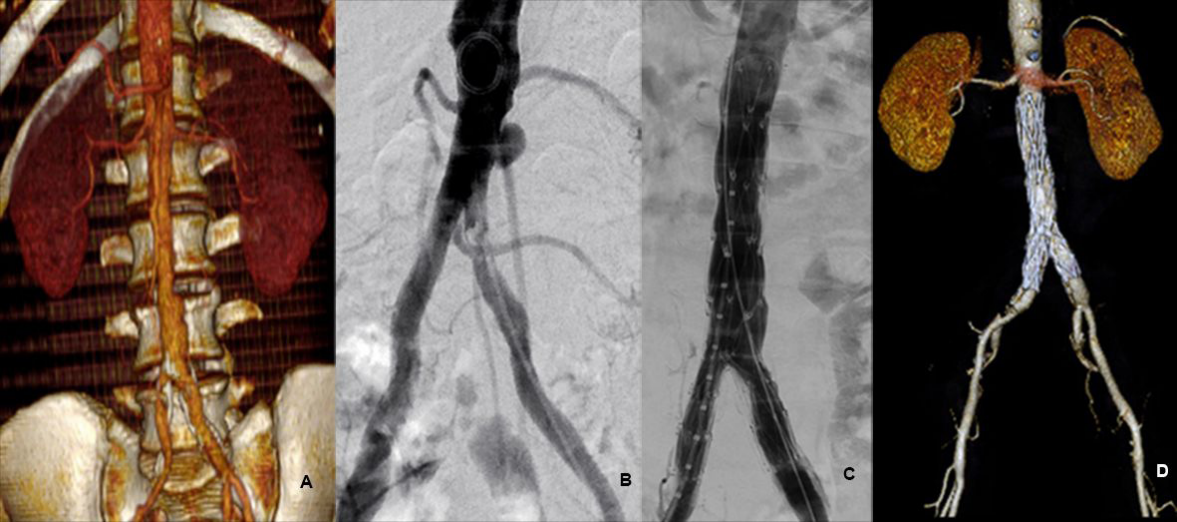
Example of PAU treated with main unibody bifurcated stent graft device only. (A) Pre-treatment CTA image; (B) Intraoperative angiography; (C) Post-treatment angiographic appearance; (D) Post-treatment control CTA.
